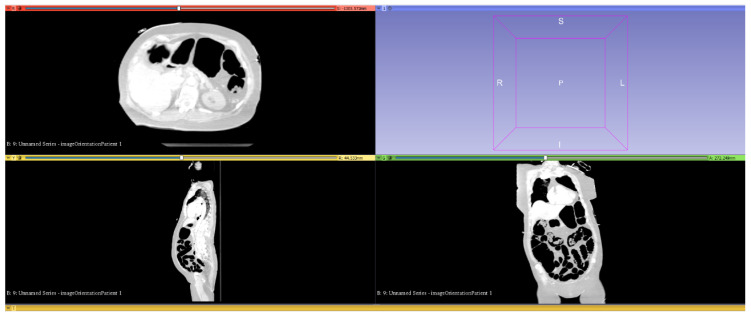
Uploaded whole-body CT scan with highlighted and segmented intestinal gas.
